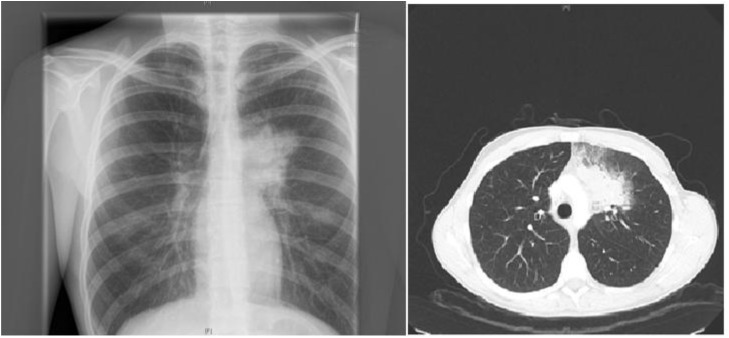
Patient’s X-ray (left) and chest-CT (right) showing a left upper lobe mass near the mediastinum resulting in a dry, non-productive cough.
